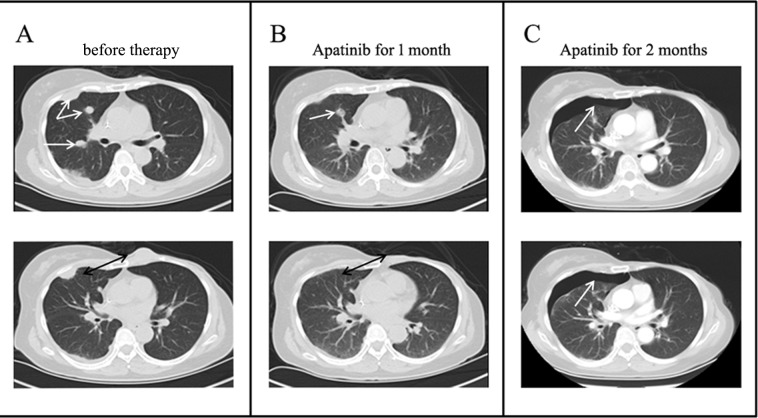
Chest CT scans before and after Apatinib therapy A. CT scans at different layers before Apatinib therapy revealed multiple metastases in right lung, pleura and chest wall; B. After 1 cycle Apatinib treatment, CT scans showed that the metastatic nodules became smaller or almost disappeared; C. After another therapy cycle, pulmonary metastasis further decreased and pneumatothorax appeared at the same site of pleura metastasis.
